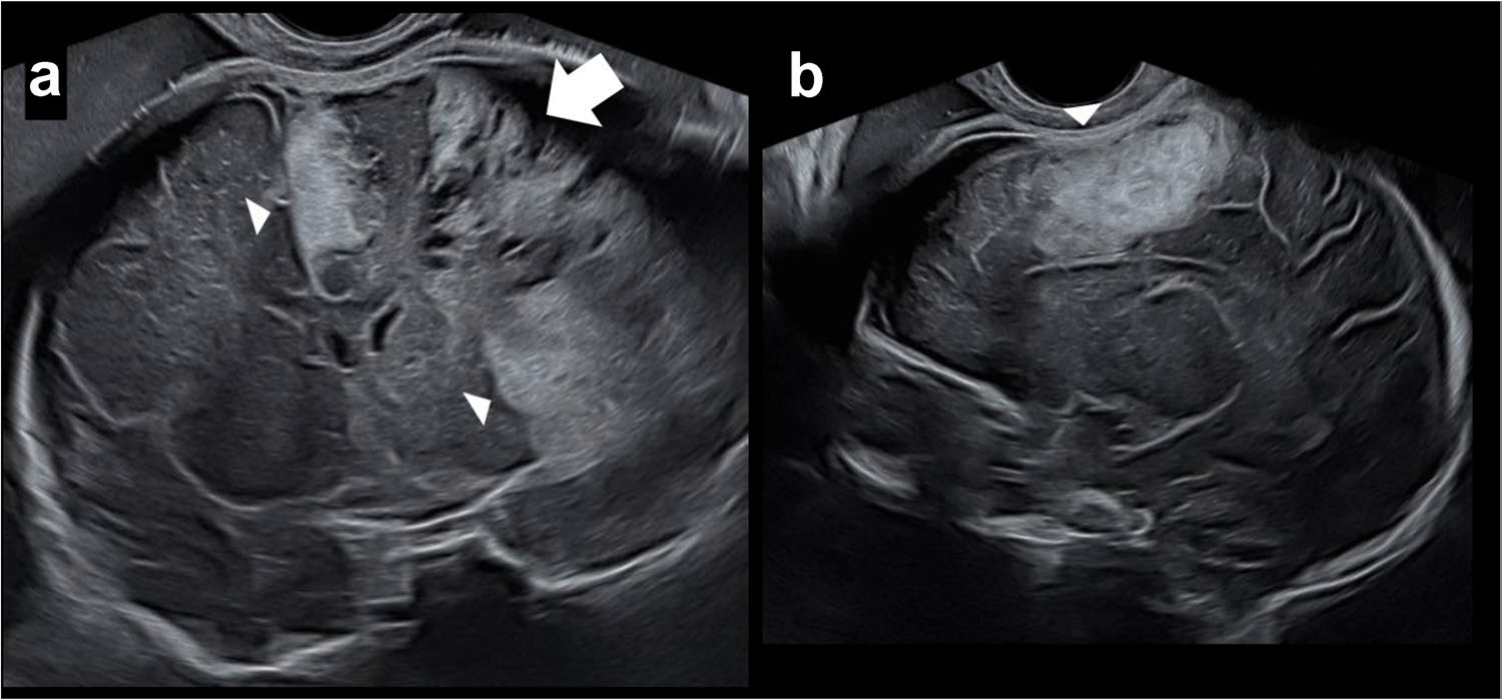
Ultrasound images of the brain in a male fetus at 32 + 4 weeks gestational age demonstrate hemorrhage. a Coronal image shows diffuse parenchyma and extra axial echogenicities, fronto-parietal lobes (*arrowhead*), with cystic evolution (*arrow*) consistent with hemorrhages at different stages. b A para-sagittal image shows echogenic parenchyma hemorrhage (*arrowhead*)
